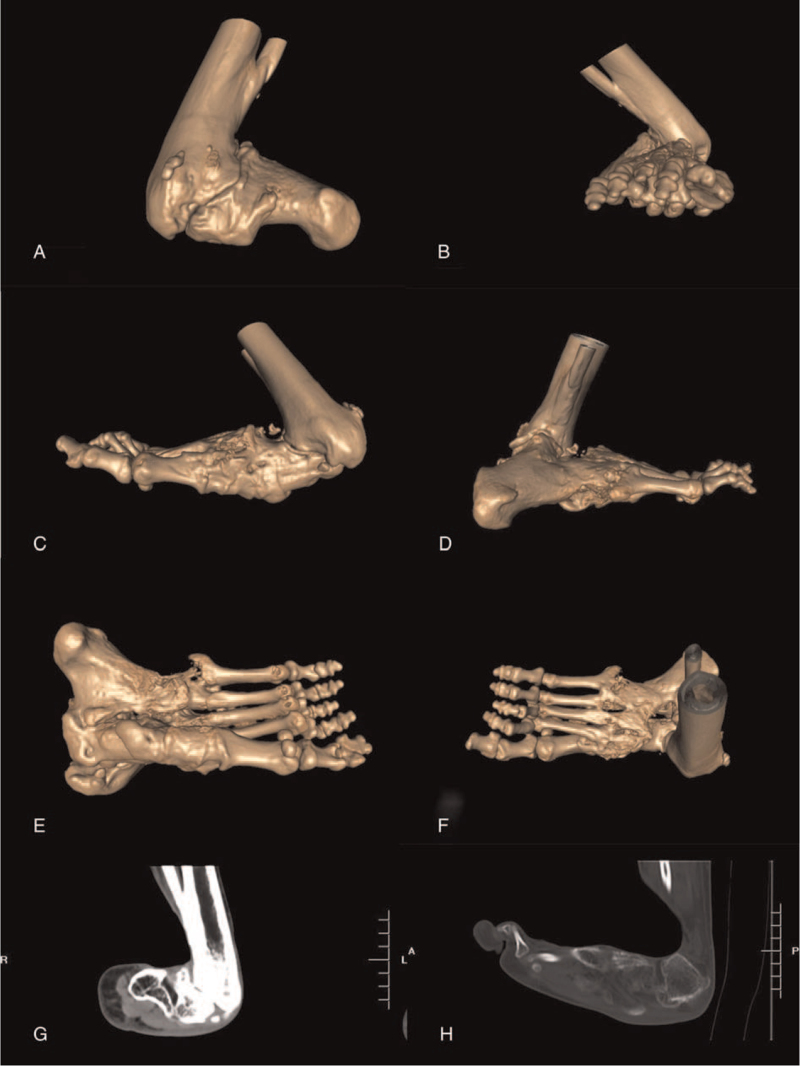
CT scan and 3-D reconstruction images before operation. (A–F) The lateral malleolus was disappeared and the inferior tibiofibular joint was deformed fusion. Tarsometatarsal, talocalcaneonavicular, talocalcaneal and calcaneocuboid joints were bony fusion. The arch of the foot was collapsed. (G and H) Inferolateral misdirection of tibiotalar joint surface and abnormal shapes of tarsal bones, calcaneus and talus.
